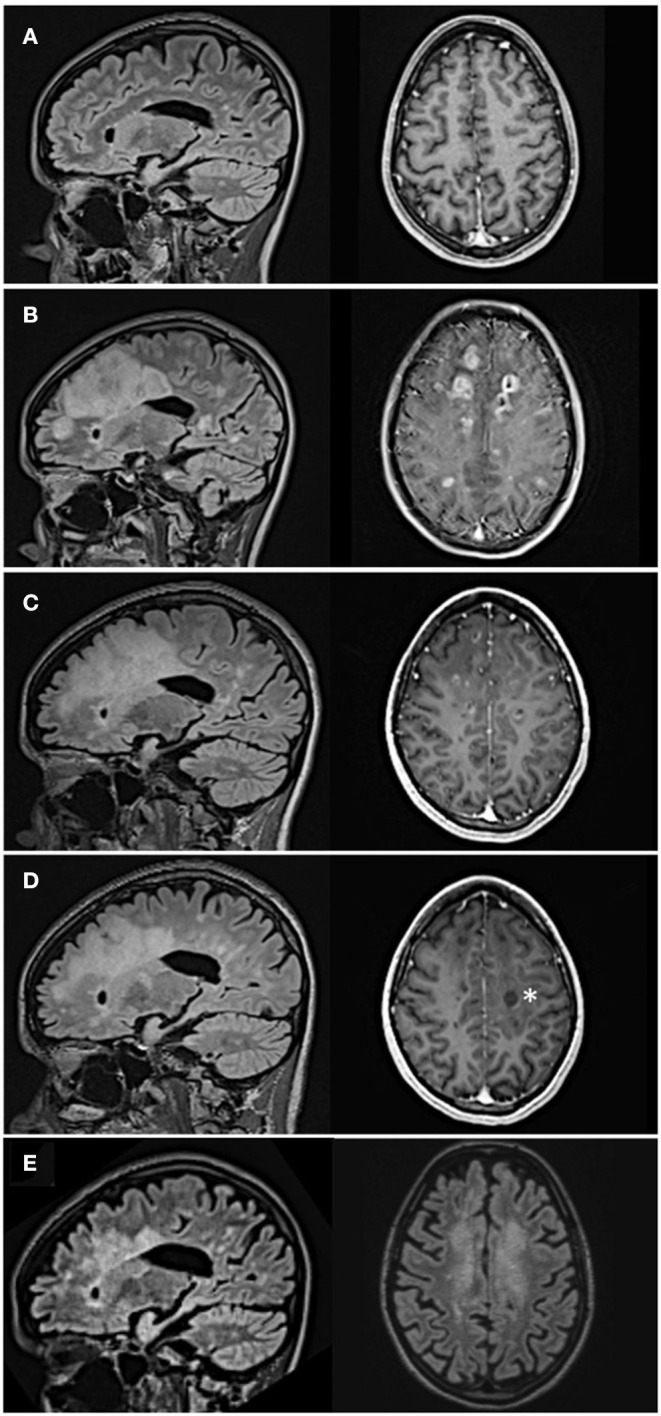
MR-imaging during disease course. Sagittal FLAIR- and axial T1 gadolinium sequences. (A) Moderate lesion load under fingolimod treatment. (B) Frontoparietal tumefactive lesions with disseminated Gadolinium-enhancement 6 weeks after discontinuation of fingolimod. (C) Remittent Gadolinium-enhancement after four cycles of plasmapheresis. (D) Further remission at re-admission 2 weeks after the last cycle of plasmapheresis; asterisk indicates active lesion. (E) Lesions decreasing in size 6 months after ocrelizumab initiation (only axial FLAIR available).
